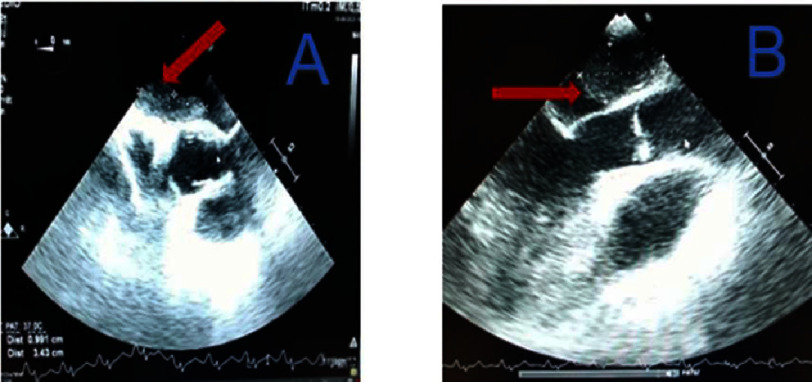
Transesophageal echocardiography revealing a myxoma of the left atrium (arrow) measuring 3.43 cm (A) and 4.5 cm in large diameter (B) in the left ventricular outflow tract.
